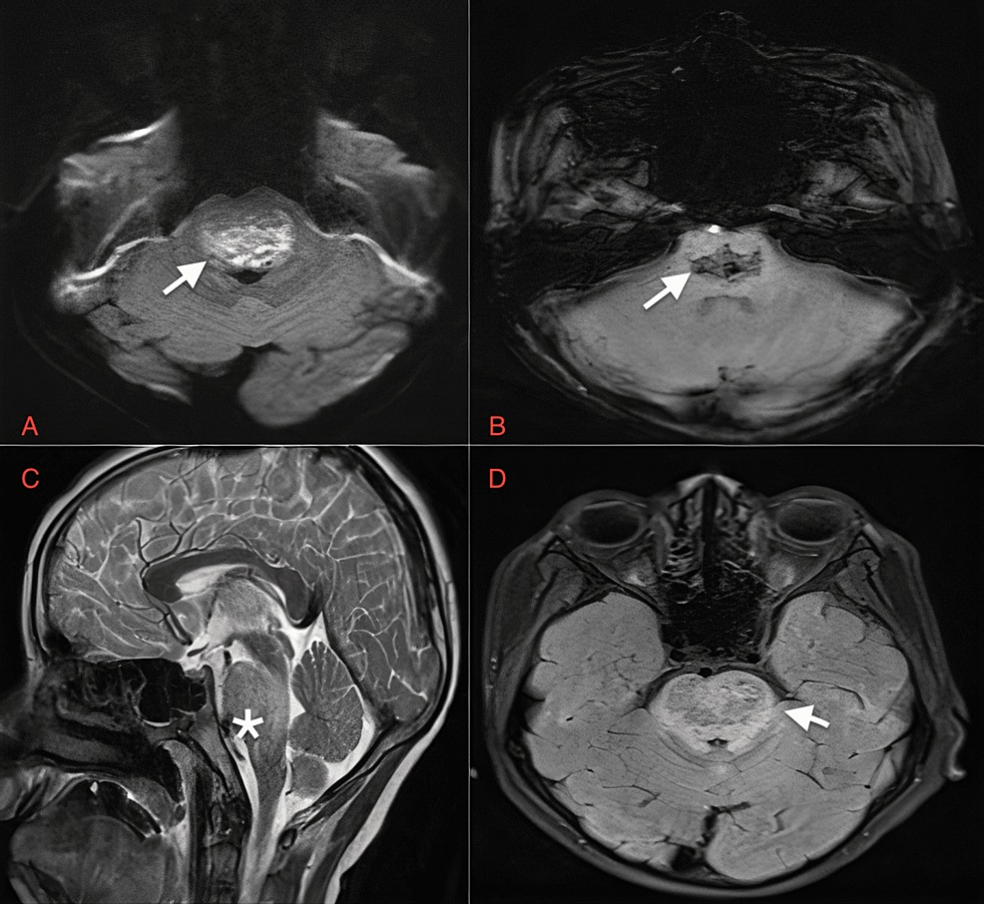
Case 8: Diffusion restriction is also seen on DWI in the pons (white arrows in A). Areas of blooming are seen on SWI (white arrow in B). Hyperintense areas are seen in pons T2W sagittal images and FLAIR images (white arrow in C and D) in a nine-year-old male patient with dengue encephalitis. DWI: diffusion-weighted image; SWI: susceptibility-weighted image; T2W: T2-weighted image; FLAIR: fluid-attenuated inversion recovery sequence
